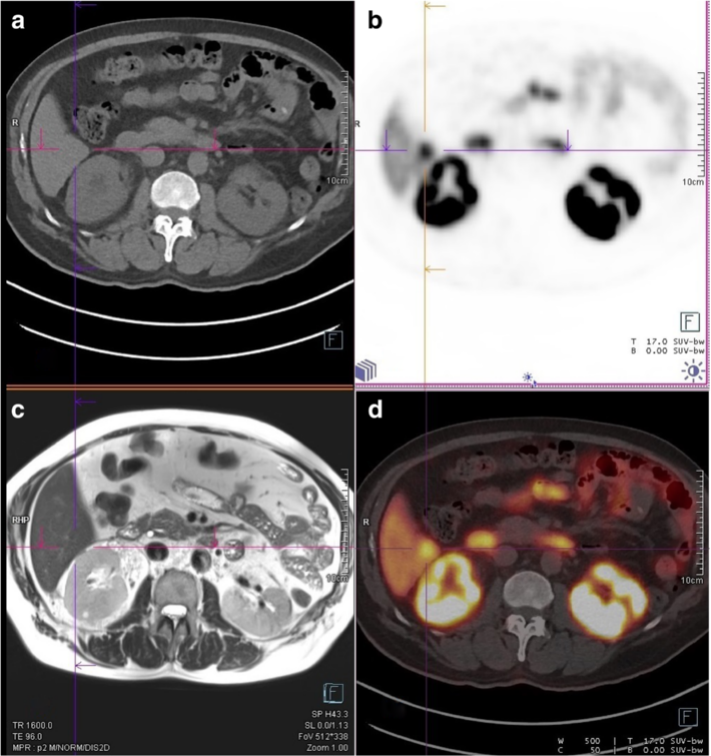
Comparison of non-contrast CT, MRI and PSMA PET in subject 2. a Non-contrast CT or c non-contrast T1 sequence of MRI did not reveal a lesion in the right lobe of the liver. On b and d which are PSMA PET images, a focal liver lesion was identified with SUVmax of 15.3 and dimensions of 17 × 13 × 14 mm. d Fused PET and CT images. The patient had moderate renal impairment and contrast allergy, prohibiting intravenous contrast with MRI or CT
